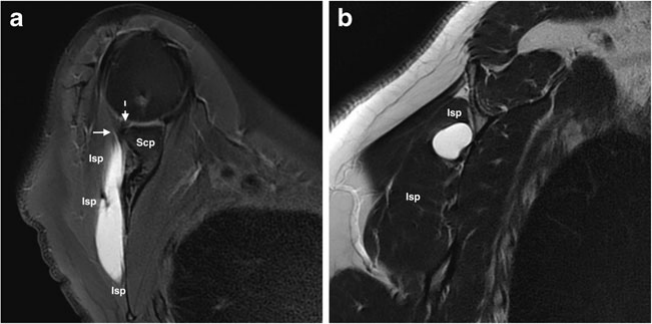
a, b. Intramuscular infraspinatus cyst in a 58-year-old woman with a known partial-thickness supraspinatus tear, presenting with exacerbated posterior right shoulder pain during elevation and external rotation. Axial FS T2-weighted MRI (a) shows a teardrop-shaped homogeneously hyperintense subaponeurotic intramuscular lesion along the posterior surface of the scapula. Note its thin extension toward the musculotendinous junction (arrow). The glenoid labrum (dashed arrow) seems preserved. A sagittal section (b) better demonstrates the location of this lesion within the infraspinatous muscle. Scp, scapula; Isp, infraspinatous
